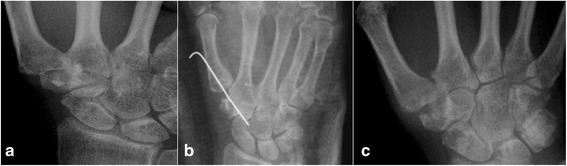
a Preoperative radiographic images demonstrating Eaton stage III osteoarthritis of the right thumb carpometacarpal joint. b Immediate postoperative radiograph showing a K-wire used for fixation across the thumb carpometacarpal joint. c Radiographs obtained at 24 months after surgery showing a stable thumb carpometacarpal joint
